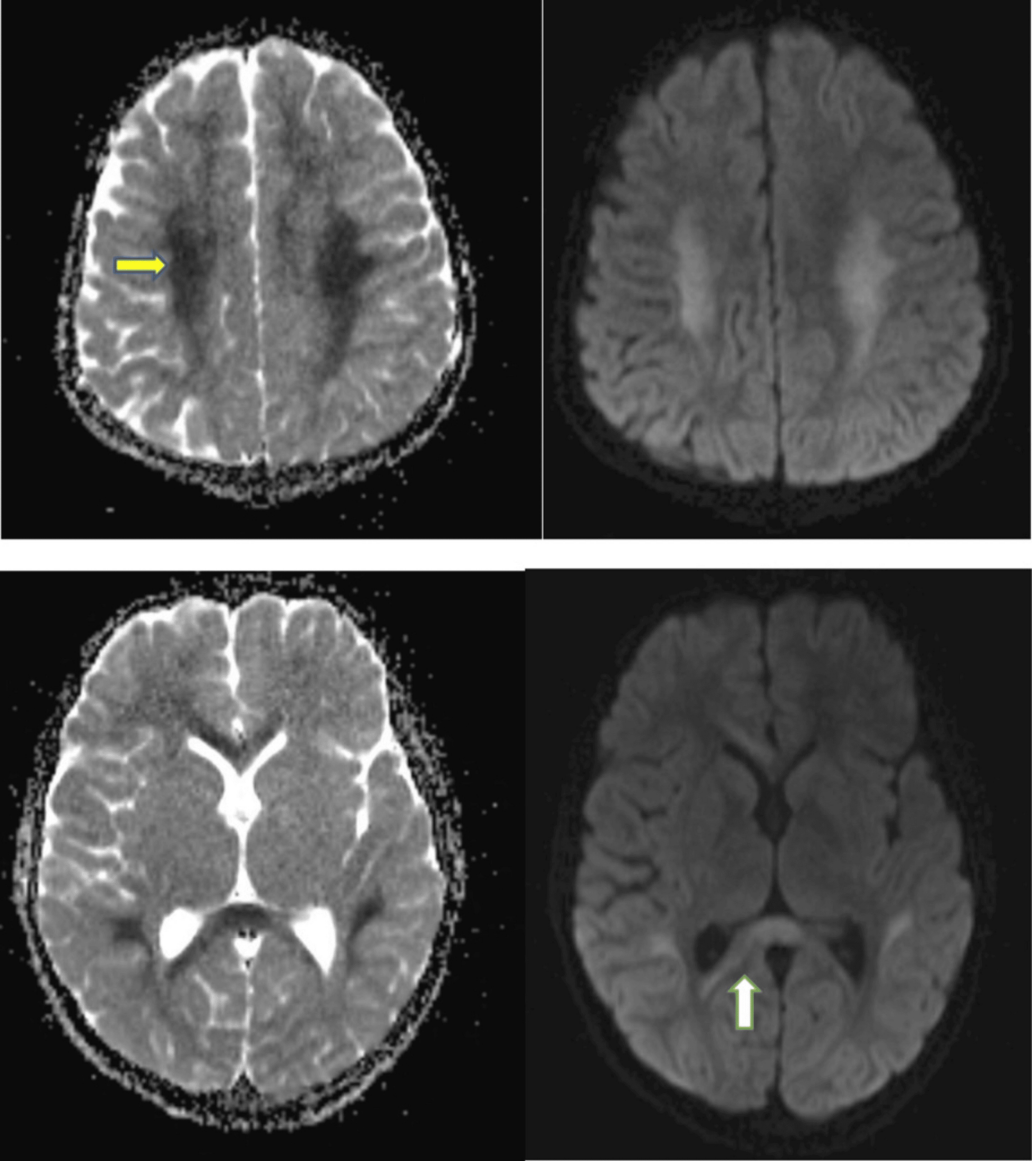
MRI ADC axial section images of a patient in our study showing "involvement of corpus callosum predominantly splenium" in a case of MERS caused by EBV MRI: magnetic resonance imaging, ADC: apparent diffusion coefficient, MERS: mild encephalitis/encephalopathy with reversible splenium lesion, EBV: Epstein-Barr virus
